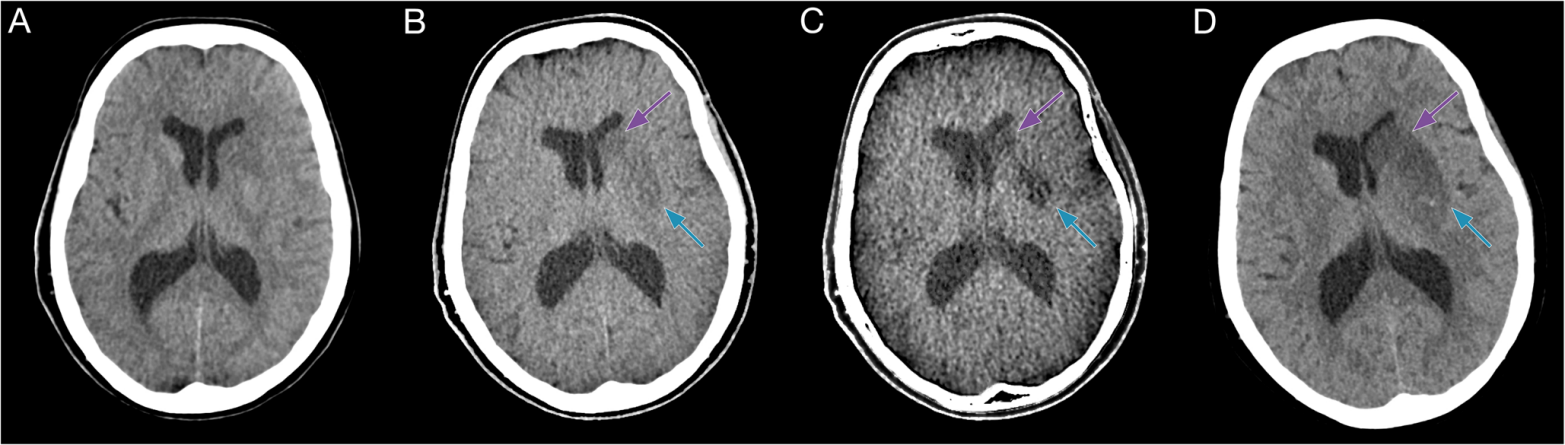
Example of ischaemic lesions in the caudate nucleus (violet arrow) and putamen (blue arrow) in the BW (A), VNC (B) and EM (C) series. In the BW (A) and VNC (B) series, the lesions are not clearly visible, however in the EM the hypodense infarctions can be clearly identified. Follow-up CT with infarction delineation (D)
